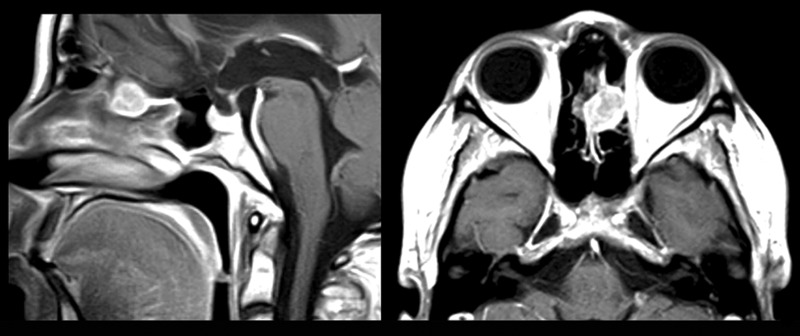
Preoperative MRI demonstrating lesion penetrating the anterior skull base Tumor involves left ethmoid roof and extends intracranially
